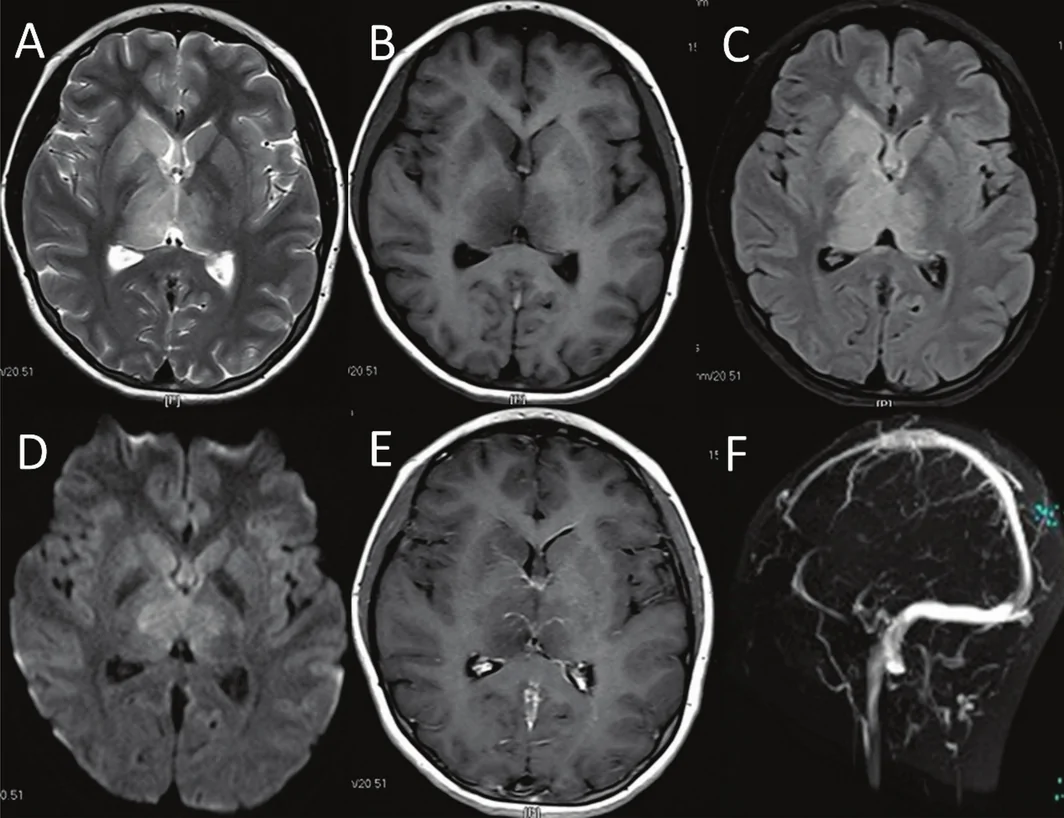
Hyperintense signals are observed in the bilateral thalami, right corpus callosum, and caudate nucleus on T2WI (A), FLAIR (C), and DWI sequences (D), with hypointense signals on T1WI (B). No contrast enhancement is seen on post‐contrast imaging (E). MRV shows nonvisualization of the straight sinus (F).
